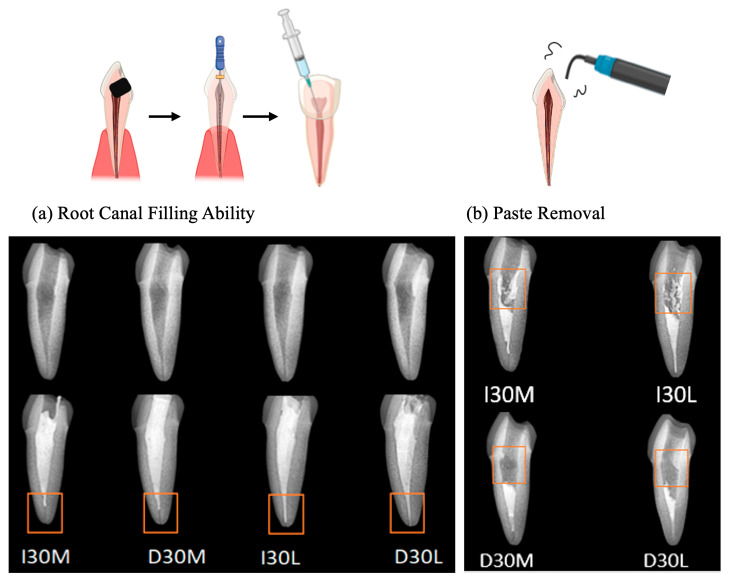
Usability test of NaI-based paste compared to iodoform-based paste. (a) Postoperative radiograph immediately after root canal treatment. (b) Postoperative radiograph after removing filling material using an ultrasonic scaler.
